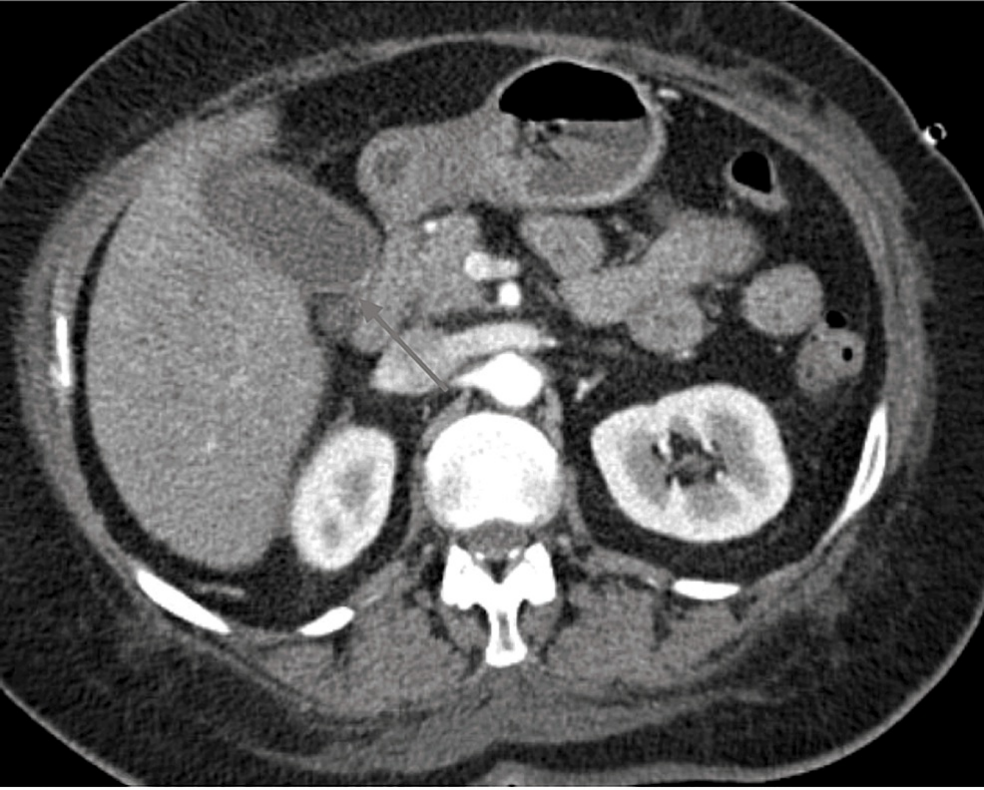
CT of the abdomen Red arrow pointing at the gallbladder with mild surrounding pericholecystic fluid. The gallstones are not seen in this image CT: computed tomography
